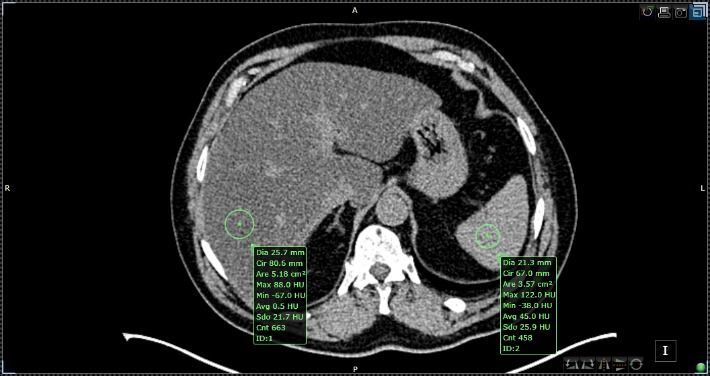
Liver steatosis semi-quantitative assessment with Siemens 16-slices-unenhanced CT with the region of interest (ROI) placement in the liver and spleen parenchyma
